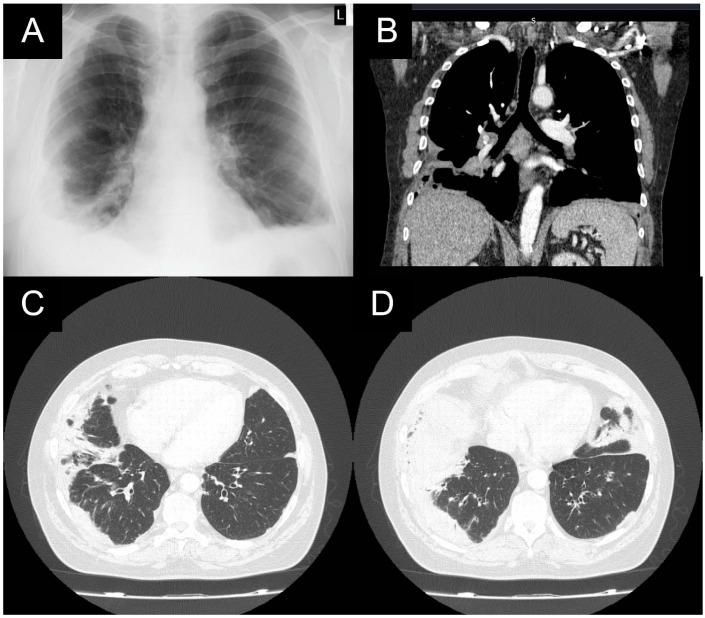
X-ray: coronal plane (A) from 2016; CT scan: coronal plane (B) and axial plane (C,D) from 2016. Extensive peripheral parenchymal infiltrates in both lower lobes, which are not typical for common pneumonia, in addition to mediastinal lymphadenopathy.
